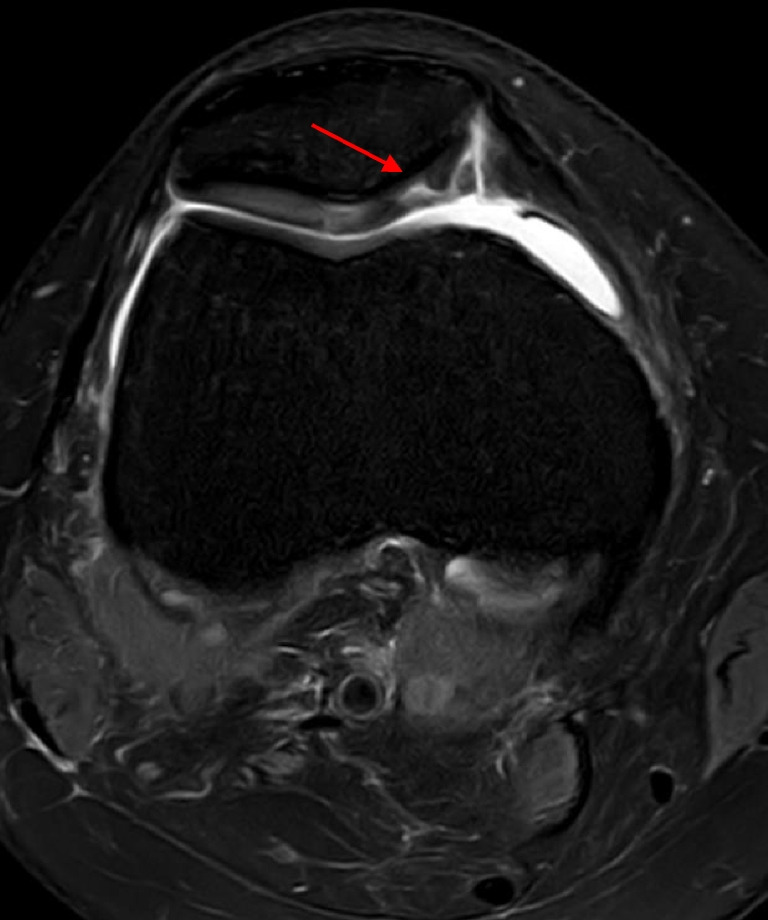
Full-thickness cartilage tear on the medial facet of the patella (arrow) with delamination of the adjacent cartilage on axial proton density fat saturation magnetic resonance imaging sequence (right knee)
